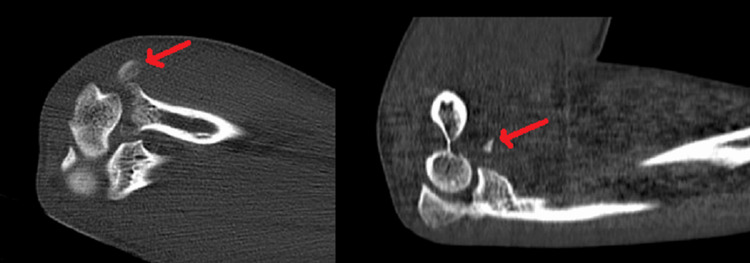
CT scan of the elbow joint showing fracture of the radial head and avulsion fracture of the coronoid (marked with red arrows) CT, computed tomography
